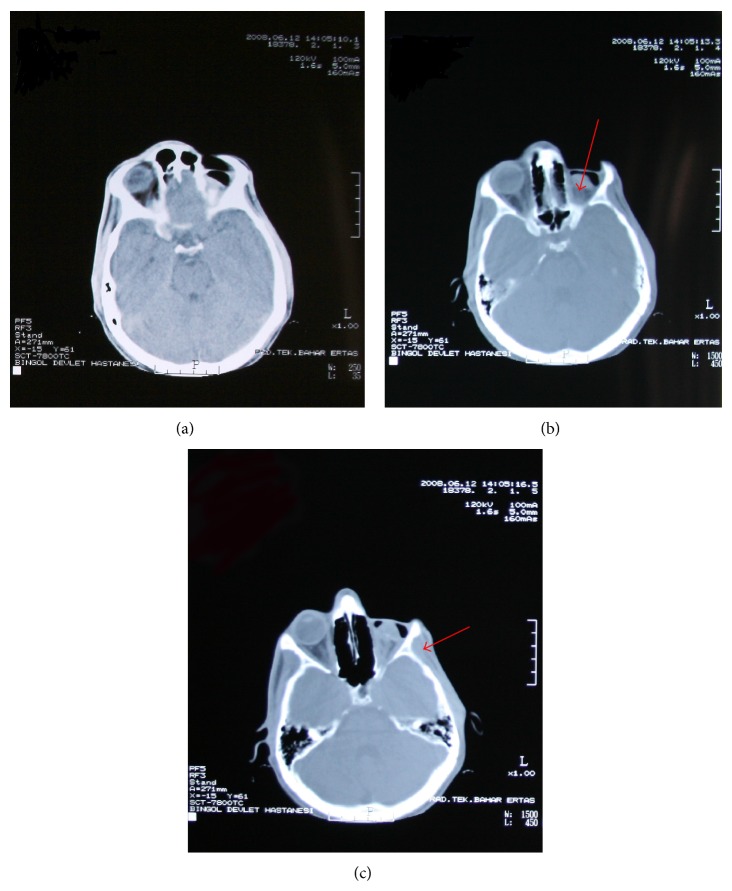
Axial computed tomography images of the head. (a) The bilateral hemispheres are normal in appearance. (b) Atrophy of the left retrobulbar fat tissue and phthisis of the eyeball (arrow) are evident. (c) The left temporal muscle (arrow) is atrophic.
